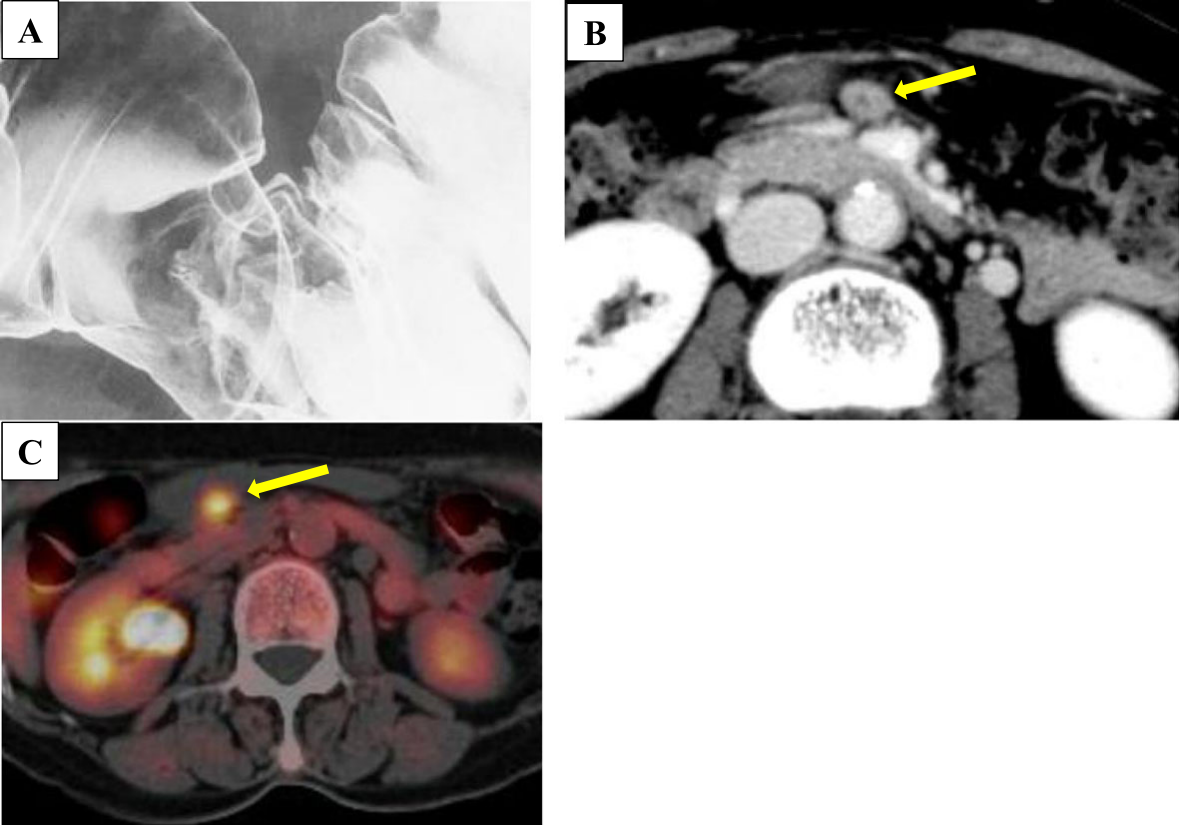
a Barium enema examination revealed a trapezoid-formed change at the hepatic flexure of the transverse colon. b Abdominal enhanced CT showed an enlarged intermediate lymph node (arrow). c PET/CT revealed an abnormal accumulation of SUVmax 3.8 on the intermediate lymph node (arrow).
